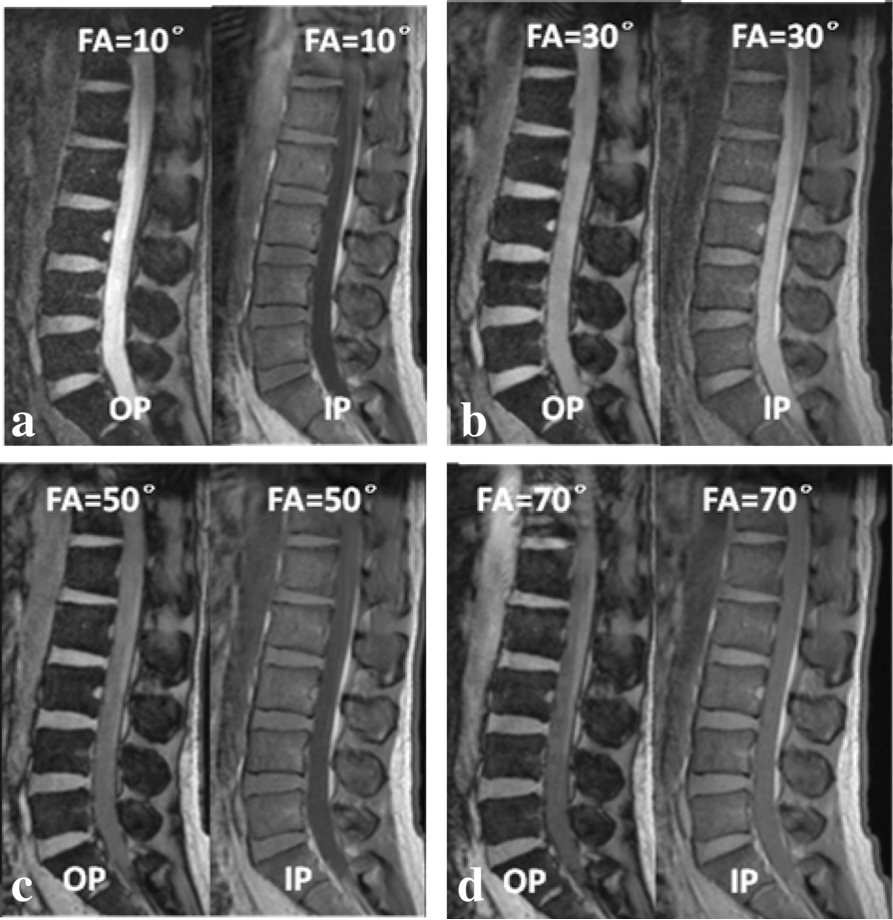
Representative images of chemical shift MR imaging in different flip angles (FA, flip angle). (a) in-phase and opposed-phase images acquired when flip angle = 10°; (b) in-phase and opposed-phase images acquired when flip angle = 30°; (c) in-phase and opposed-phase images acquired when flip angle = 50°; (d) in-phase and opposed-phase images acquired when flip angle = 70°
